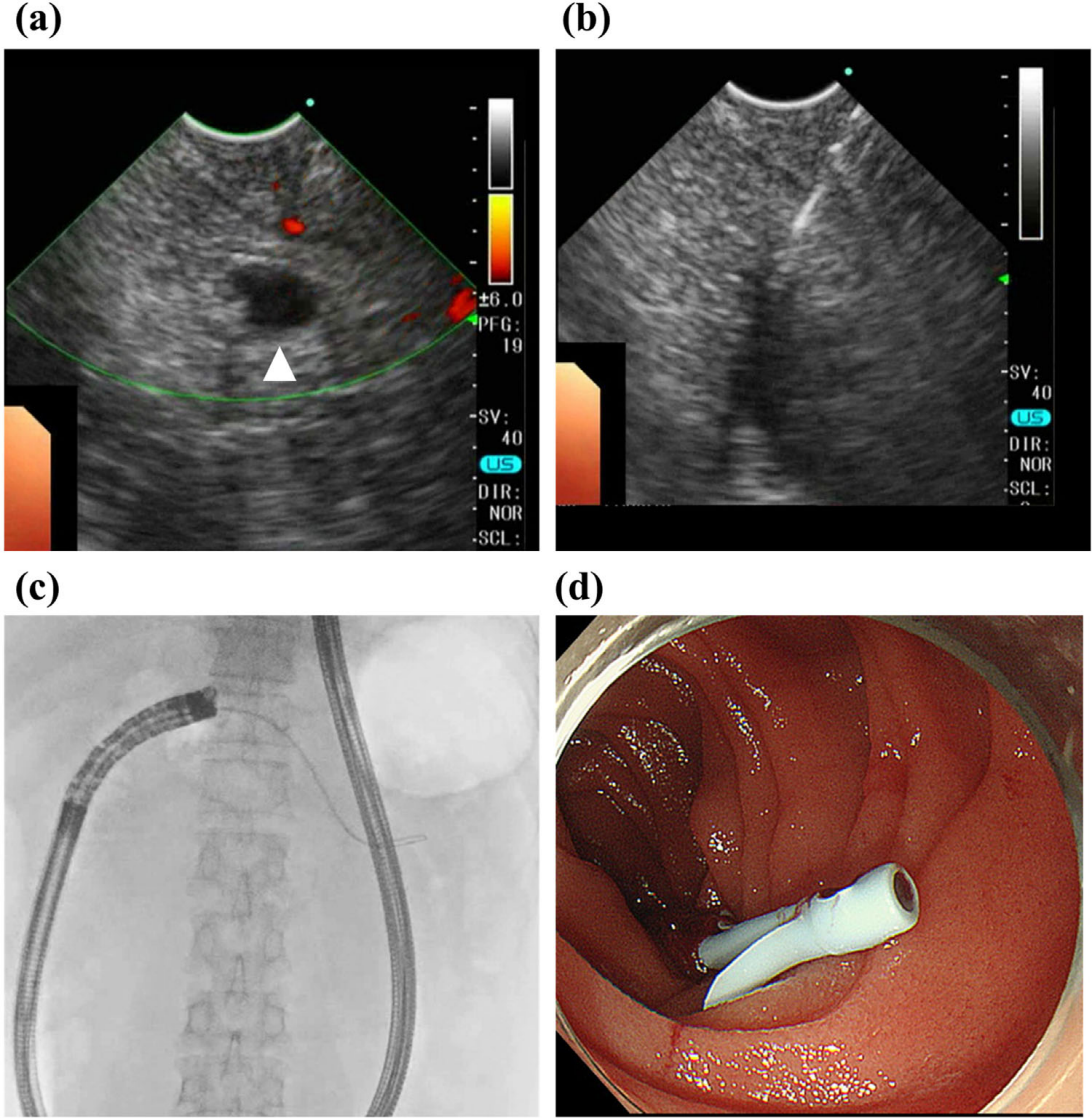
Transjejunal endoscopic ultrasound‐guided pancreatic drainage. (a) Dilated pancreatic duct (5 mm) was detected under anastomosis by endoscopic ultrasound (arrowhead). (b) The pancreatic duct was punctured by a 19‐gauge needle (EZ shot 3 plus; Olympus, Tokyo, Japan). (c,d) A 5‐Fr plastic stent was finally placed in the pancreatic duct
